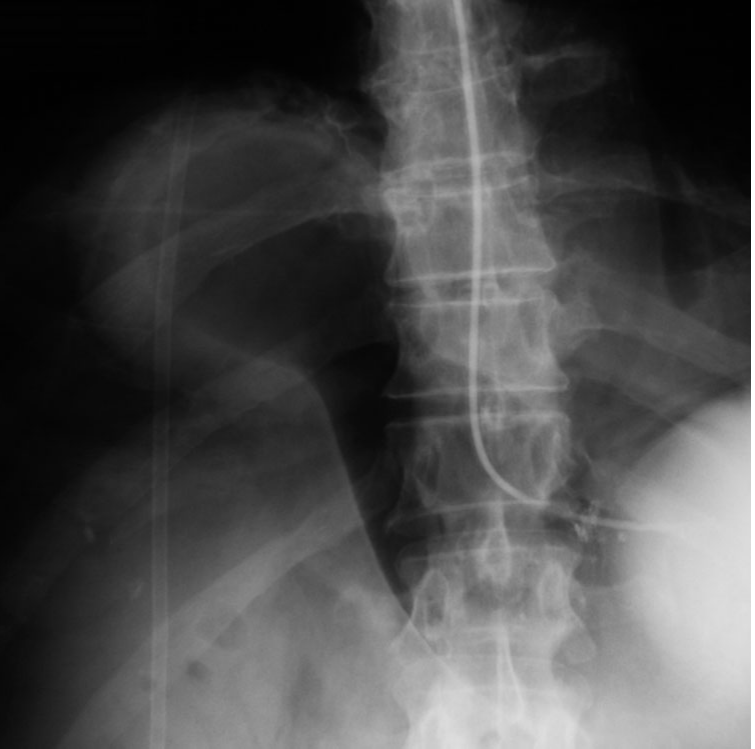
Plain x-ray showing the gastric volvulus.
